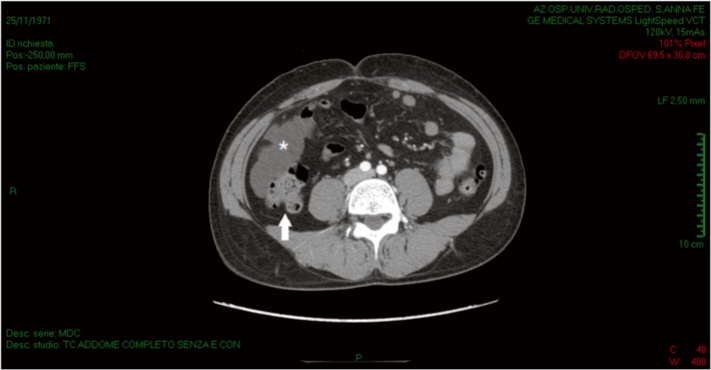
Abdominal computed tomography. Grape-like multicystic mass (*asterisk*) in the right parietocolic recess, adherent to the wall of the ascendant colon (*white arrow*) and parietal peritoneum
